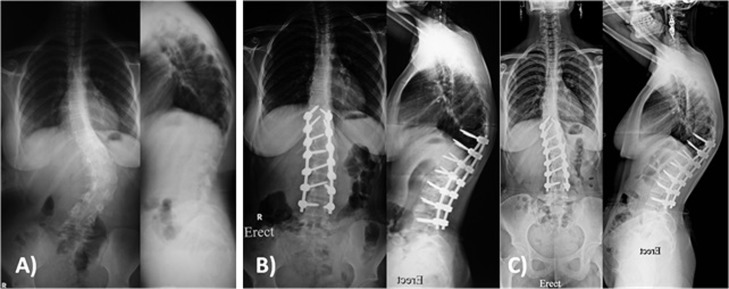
Female patient 17 years old with Lenke 5 c +. (A) Preoperative X-rays showing lumbar curve of 56° with thoracic kyphosis of 50°. (B) Postoperative X-rays showing posterior correction and fusion from T10-L4 with lumbar curve correction to 9° with thoracic kyphosis of 53° and radiological PJK. (C) Two years follow-up X-rays showing no progression of the PJK with preserved coronal correction.
